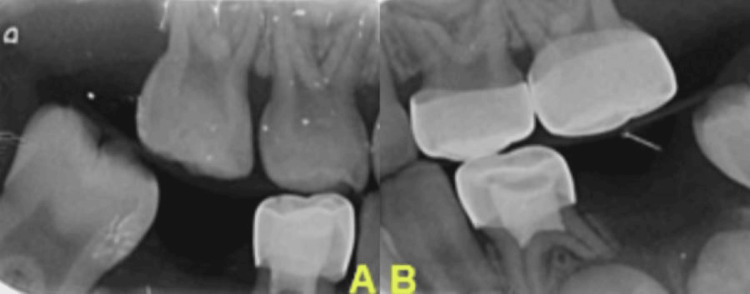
Post-operative (a) left and (b) right molar bitewing radiographs after 6 months
